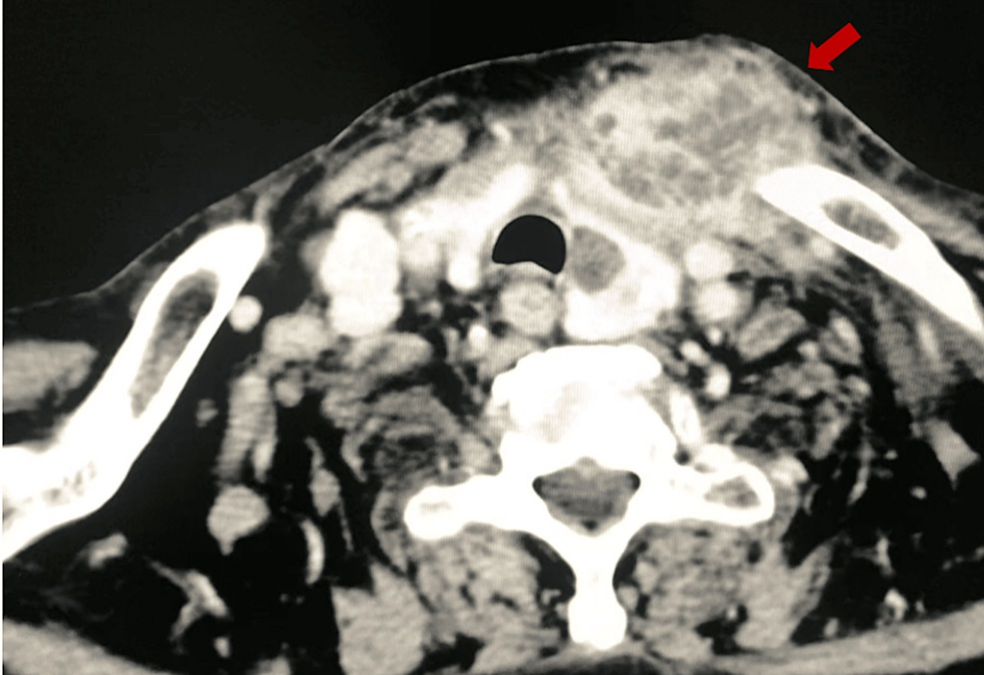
Computed tomography (axial view) reveals a multiloculated abscess impacting the right sternoclavicular joint.
